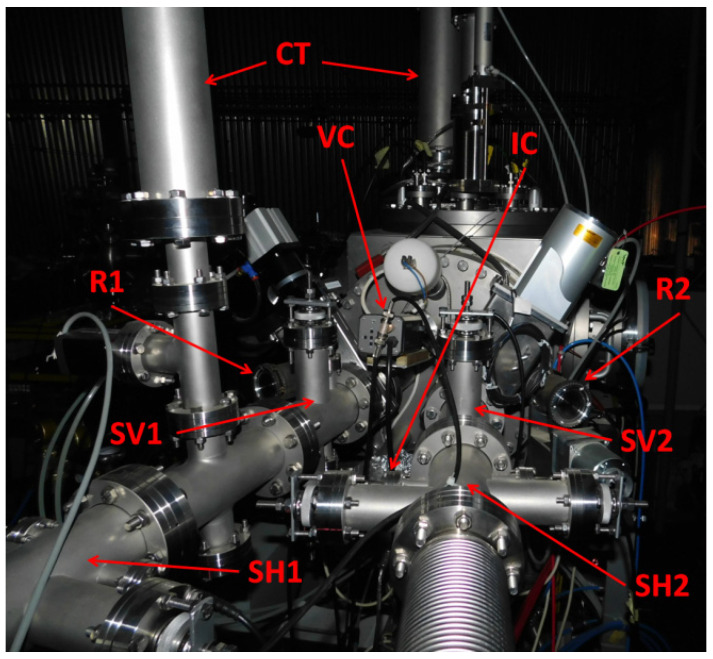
DiFU’s chamber’s front flange; CT—Liquid Nitrogen Cold Traps; R1 & R2—Magnetic Coupling Rotators for ion beam energy degraders; SH1 & SH2—Horizontal Slits; SV1 & SV2—Vertical Slits; VC—Video Camera; IC—Infra-Red Camera.
